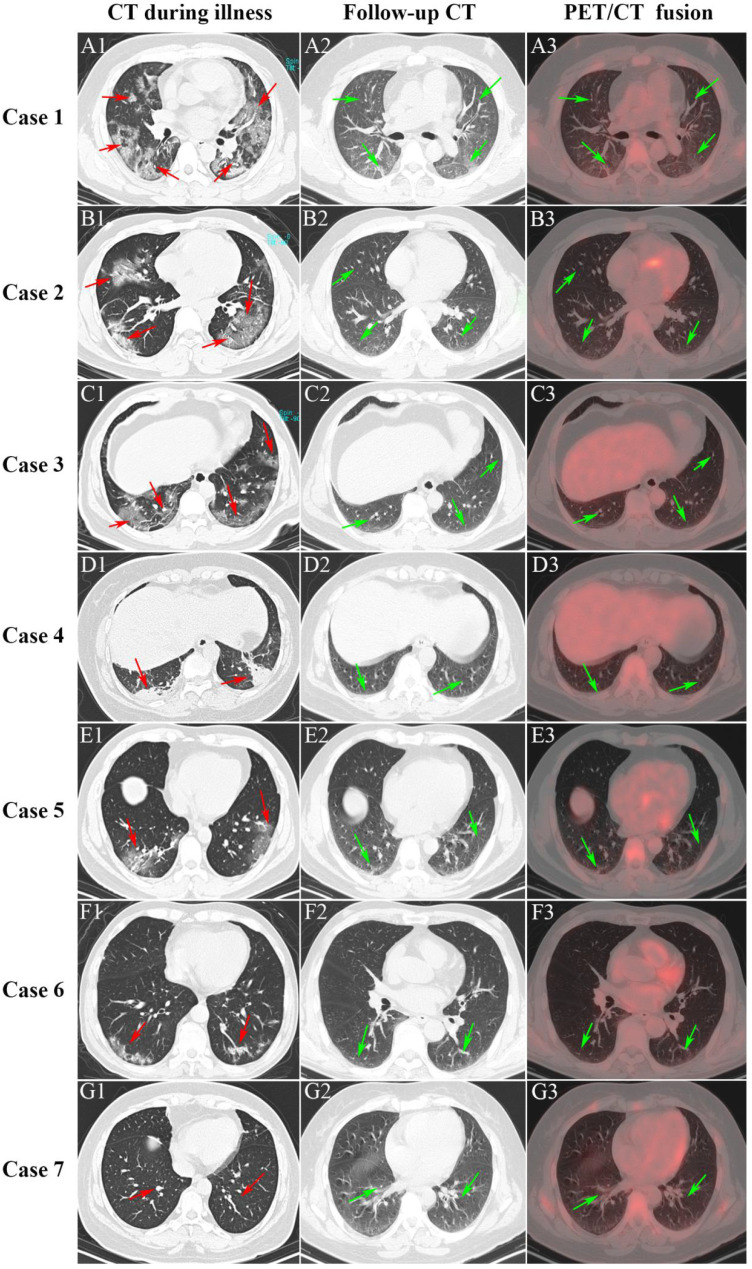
Serial CT and PET/CT demonstrated recovery of COVID-19. The first four patients (Case 1-4) suffered severe pneumonia and the last three patients (Case 5-7) suffered mild pneumonia.
